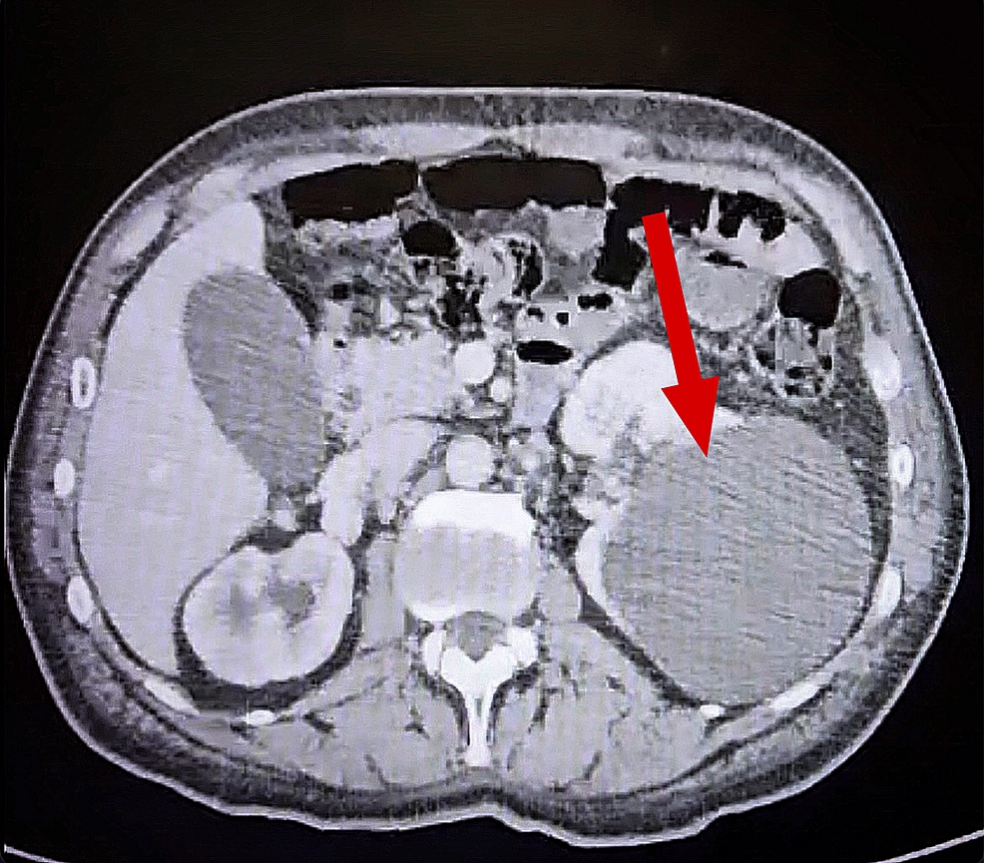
CT showing hematoma in the upper pole of the right kidney
